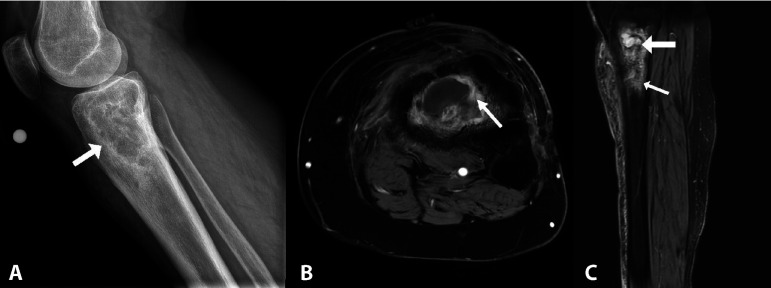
Plain film (A) demonstrating chronic tibial osteomyelitis with intraosseus abscess. MRI images from the same patient demonstrating intense peripheral enhancement consistent with a granulation layer (B, axial sequence post gadolinium contrast administration) and internal fluid signal (larger arrow) and marked peripheral edema (smaller arrow) again suggestive of intraosseus abscess (C, sagittal T2 fat-saturated sequence).
